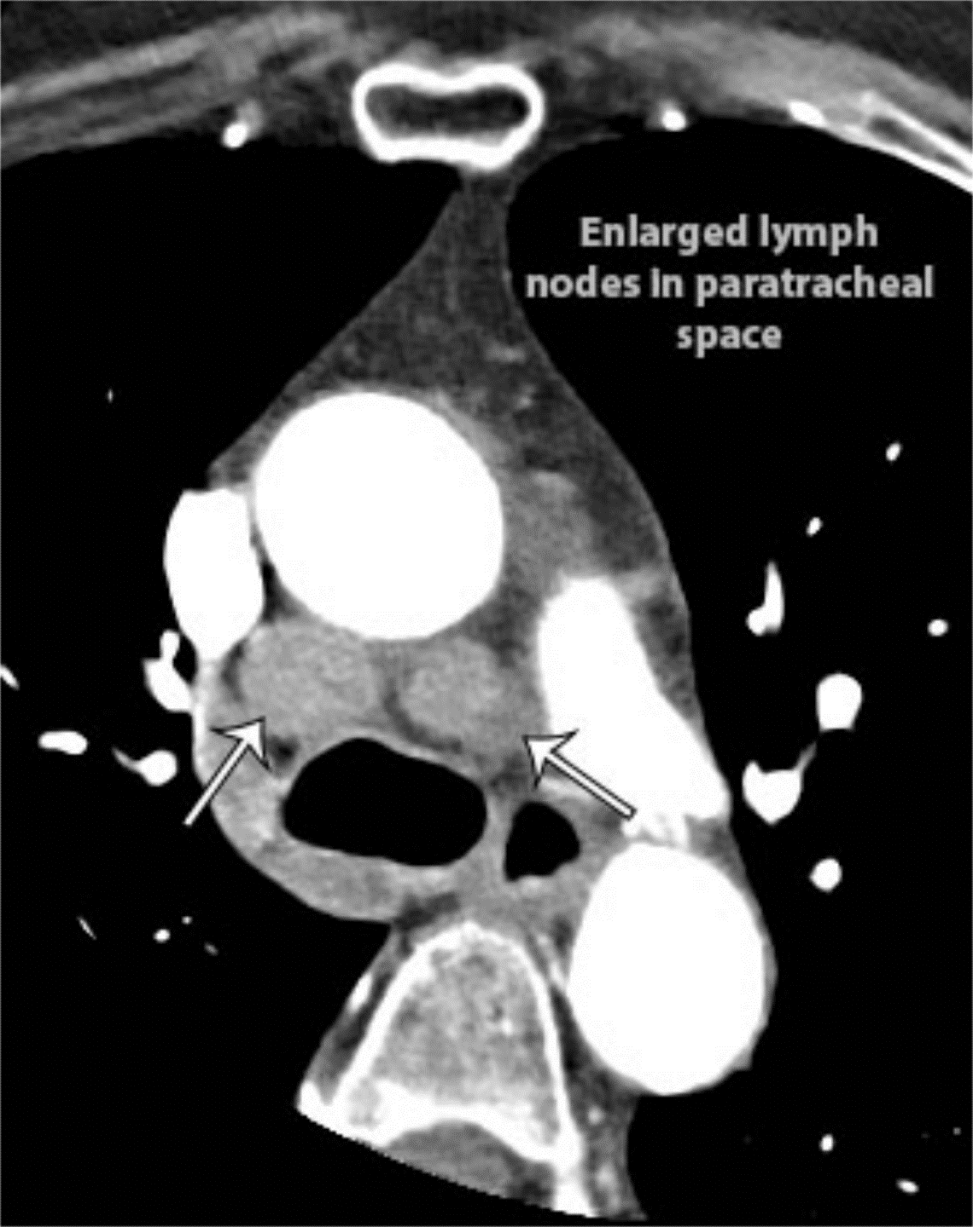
Axial coronary CT angiogram with contrast.
